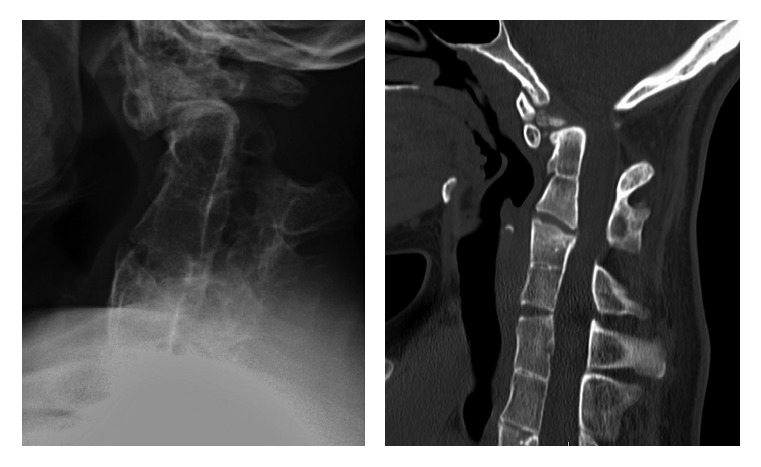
Lateral cervical spine and sagittal CT scan of the cervical vertebrae showing fusion of C2-C3, C4-C5, and C6-C7.
